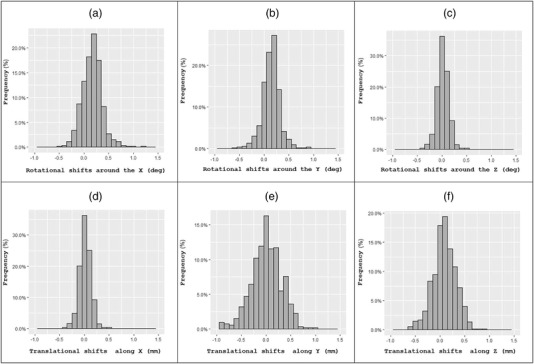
Distribution of rotational (a–c) and translational (d–f) displacements between stereotactic spaces defined by G‐frame and CBCT. In six (1.1%) cases the rotational displacement exceeded 1°. In eight (1.5%) cases the translational displacement exceeded 1 mm. CBCT, cone beam computed tomography
